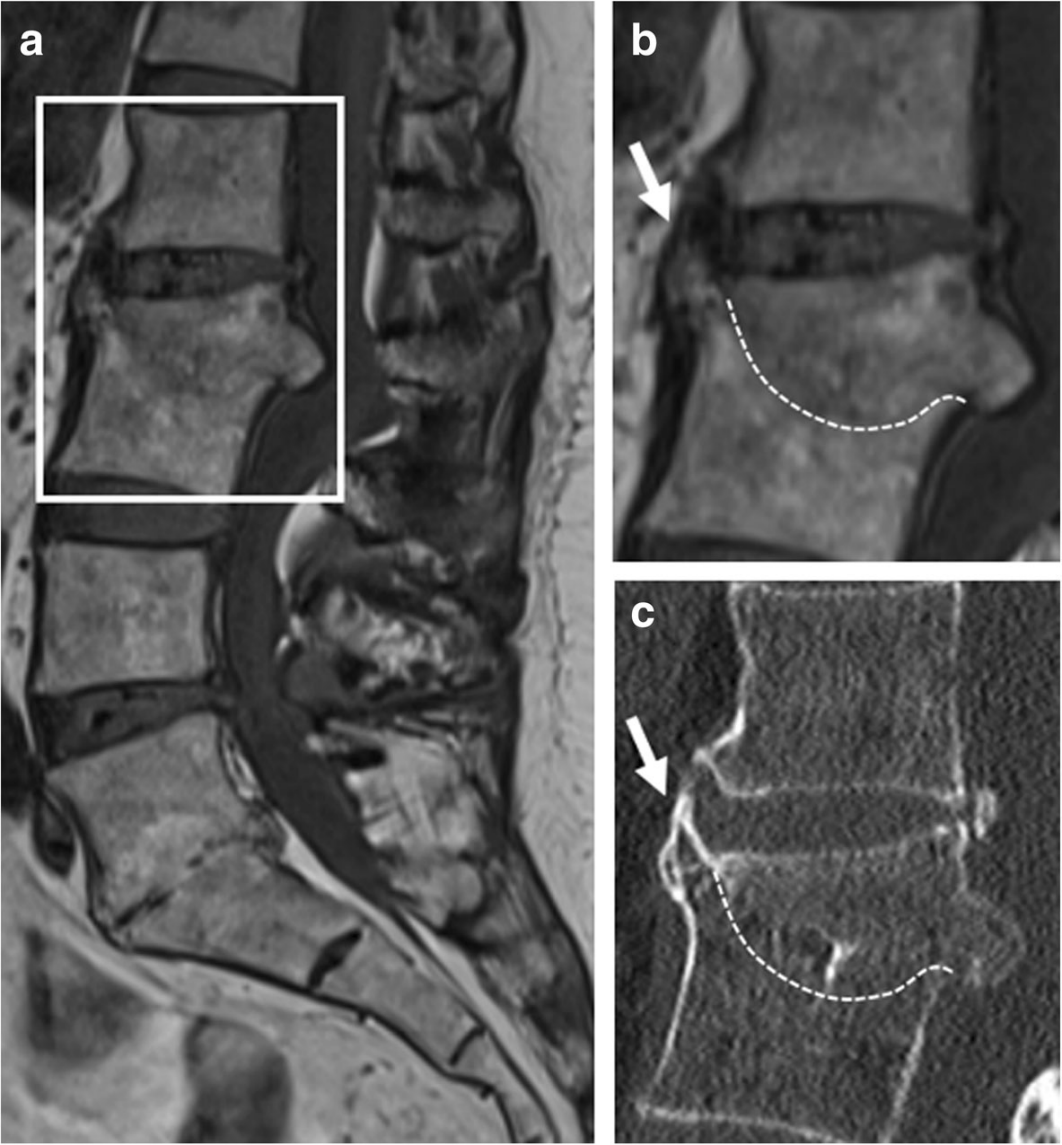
Pitfalls in imaging and diagnosis of new bone formation: sequelae of infectious spondylodiscitis. a, b Sagittal T1-weighted MR images and (c) computed tomography (CT) show vertebral fusion (dotted line) of L2-L3 after destruction of the vertebrae and intervertebral disc due to tuberculous spondylodiscitis. Note that the protrusion of the remainder of the anterior corner of the L3 mimics plump syndesmophyte formation (short arrow). L5-S1 also shows signs of vertebral fusion after destruction of the intervertebral disc, mimicking transdiscal ankylosis as seen in SpA
